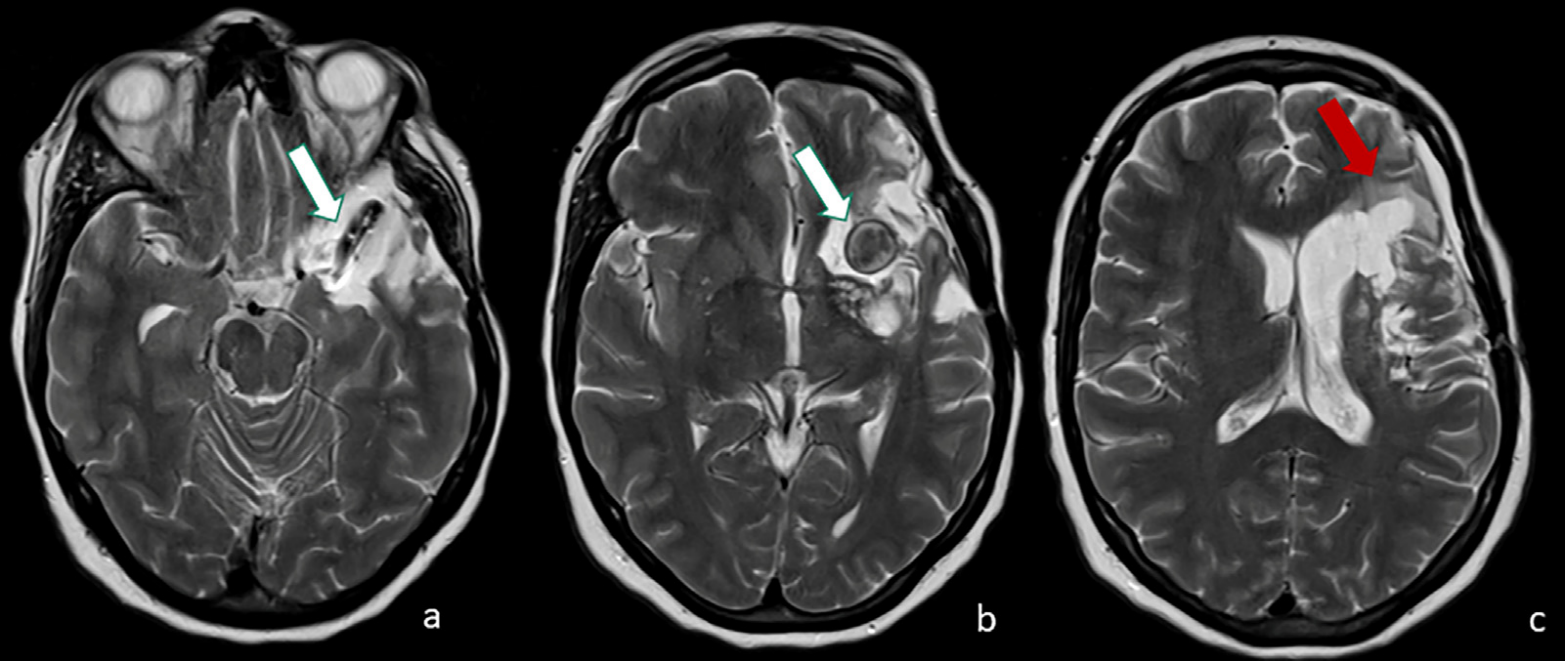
(A–C) follow-up axial T2-weighted images 10 months after the surgical treatment documented significant volumetric reduction of the residual aneurysm (A, B, white arrow) and malacic ischemic damage in the fronto-basal region (C, red arrow).
